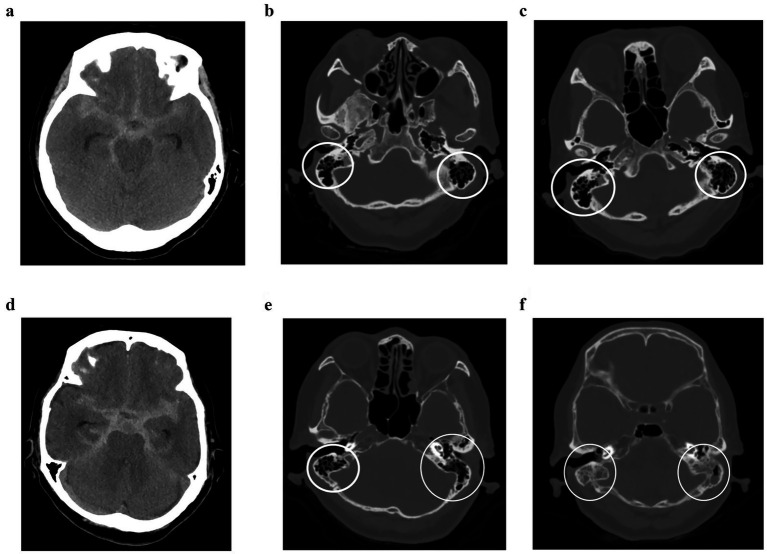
Mastoid effusion (ME) in patients with subarachnoid hemorrhage (SAH). (a–c) Case with absence of ME. A 66-year-old woman with an initial Fisher’s grade III SAH. The initial brain CT scan showed no evidence of ME. A follow-up CT scan 2 week later also showed no signs of ME (white circle). The patient’s modified Rankin Scale (mRS) score at post-operative 90 days was 0. (d–f) Case with the presence of ME. An 80-year-old woman with Fisher’s grade III SAH on the initial CT scan, with no signs of ME. Two weeks later, a follow-up CT scan revealed bilateral ME (white circle). The patient had an mRS score of 4 at post-operative 90 days.
